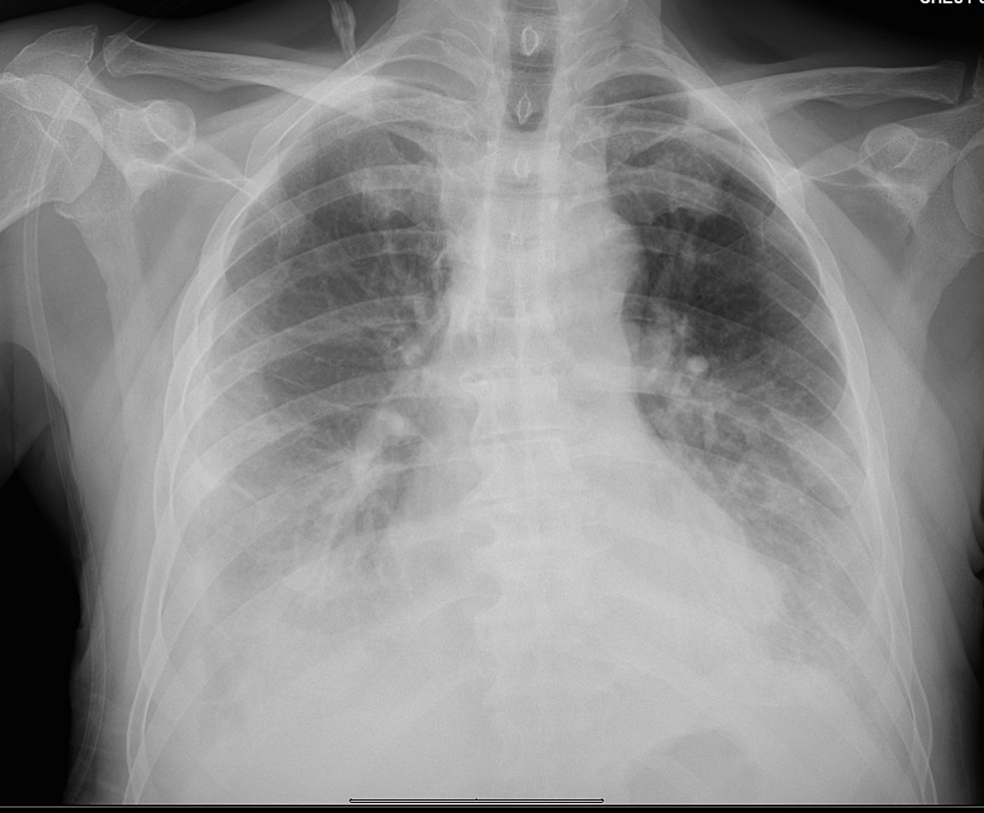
Chest x-ray showing bilateral pleural fluid with left lung fluid increase that developed about a week following the right lung pleural effusion drain
